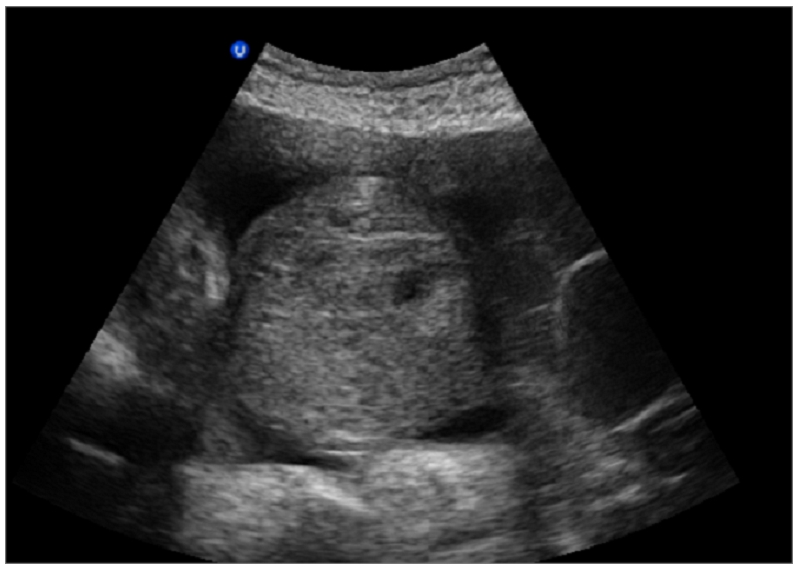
abdominal ultrasonography showing a heterogeneous mass in the uterine cavity that was difficult to distinguish from the myometrium in a patient with Ovarian hyperstimulation syndrome in a spontaneous pregnancy with invasive mole
